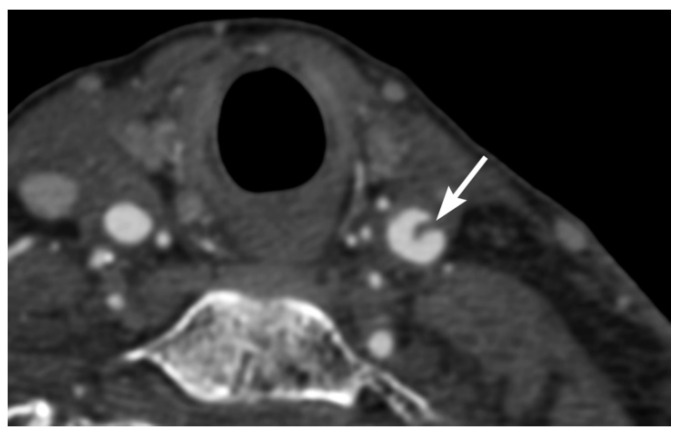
72-year-old male involved in a motor vehicle accident. Axial multidetector CT angiographic image shows a thin linear raised intimal flap in the left common carotid artery.
